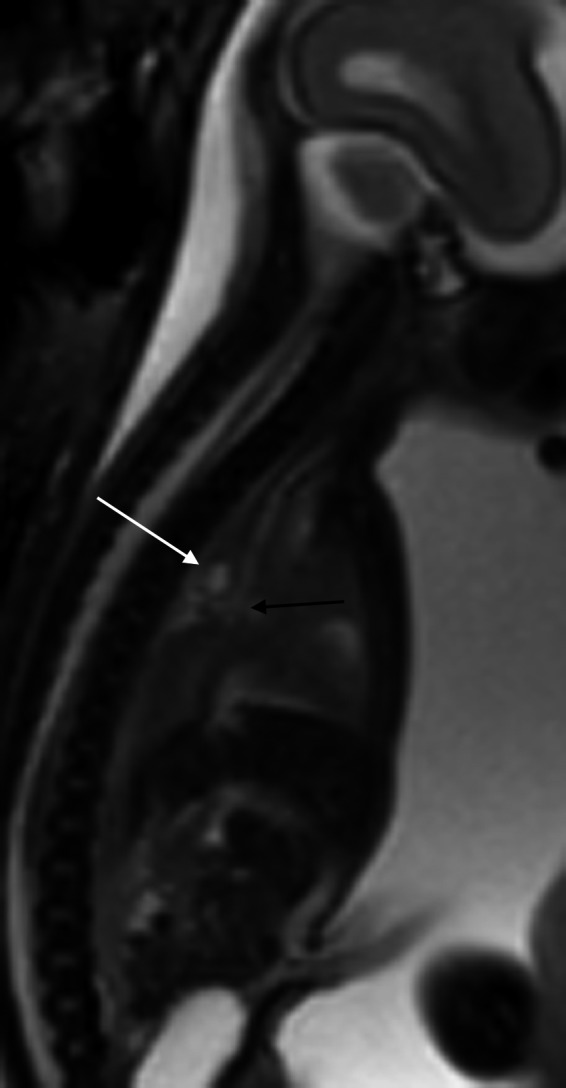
Midline sagittal SS‐FSE MRI sequence of the fetal chest and abdomen demonstrating the relationship between the carina (black arrow) and the foregut duplication cyst (white arrow)
